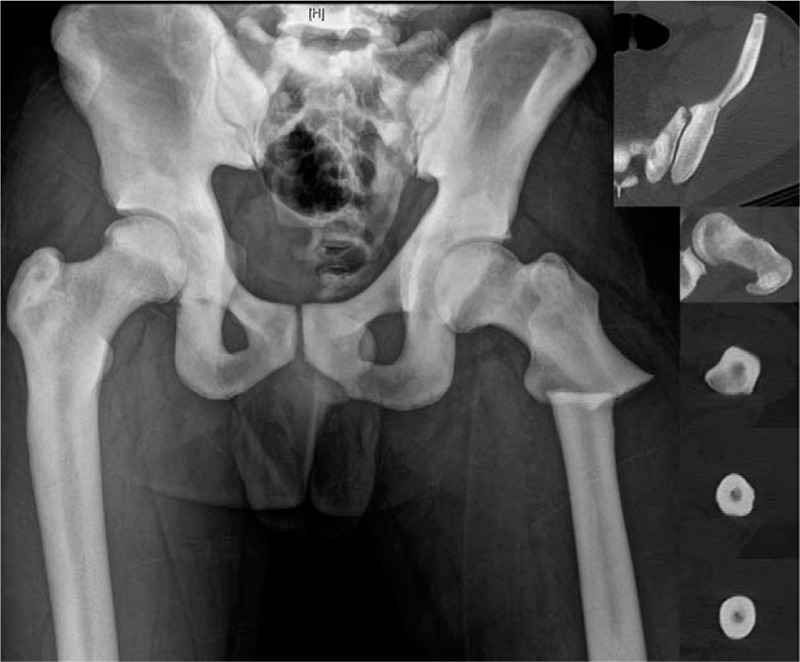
Typical radiography and computed tomography images of the pelvis and femur in a case of autosomal type II osteopetrosis. Generalized osteosclerosis with “bone-within-bone” structures are shown. Inlets, axial computed tomography images matched at the level of the femur.
